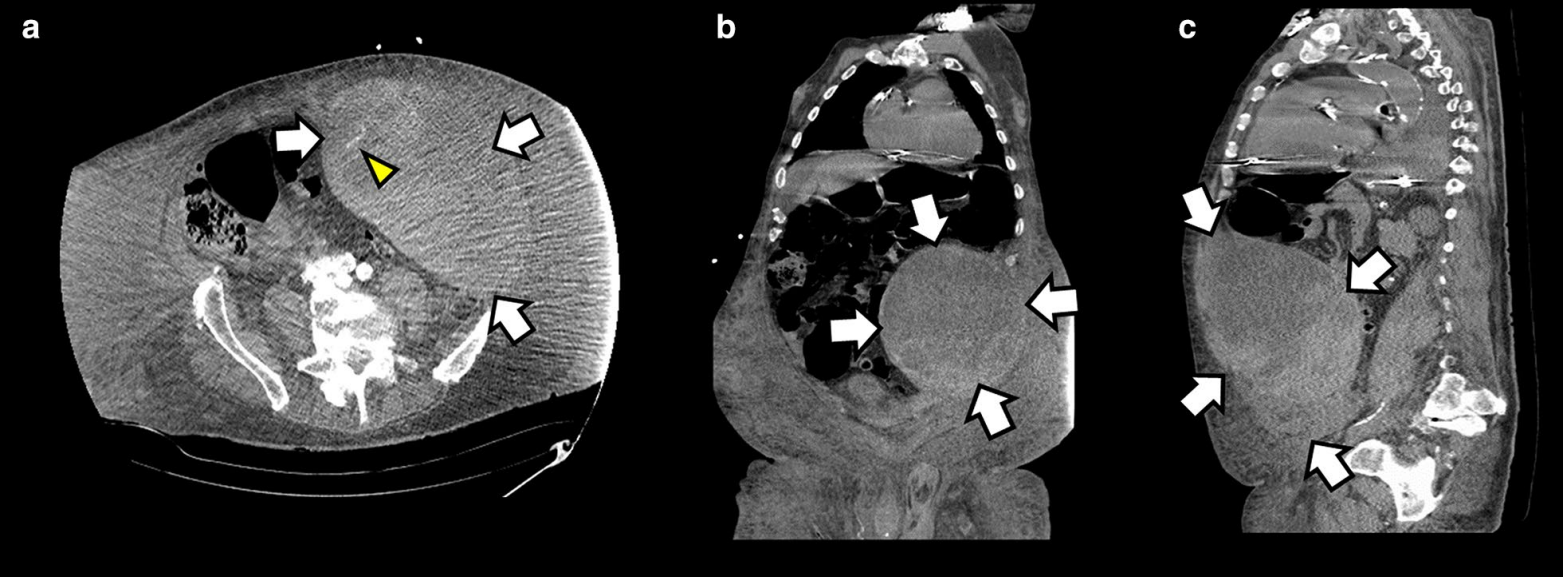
Abdominopelvic computed tomography with intravenous contrast showing a large left RSH (arrows) extending to the pelvis. Signs of active contrast extravasation are shown in a (arrowhead), indicating active bleeding; a axial plane; b coronal plane; c sagittal plane
